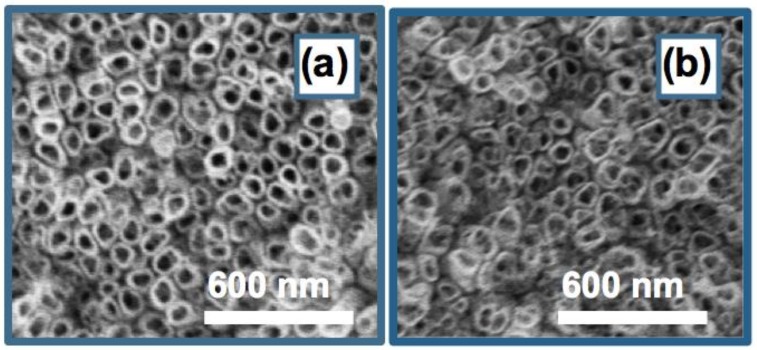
FESEM images of (a) ODPA@TNTA and (b) PFDPA@TNTA recorded after intense cavitation by ultrasonication in water for 10 min followed by additional 60 min.
